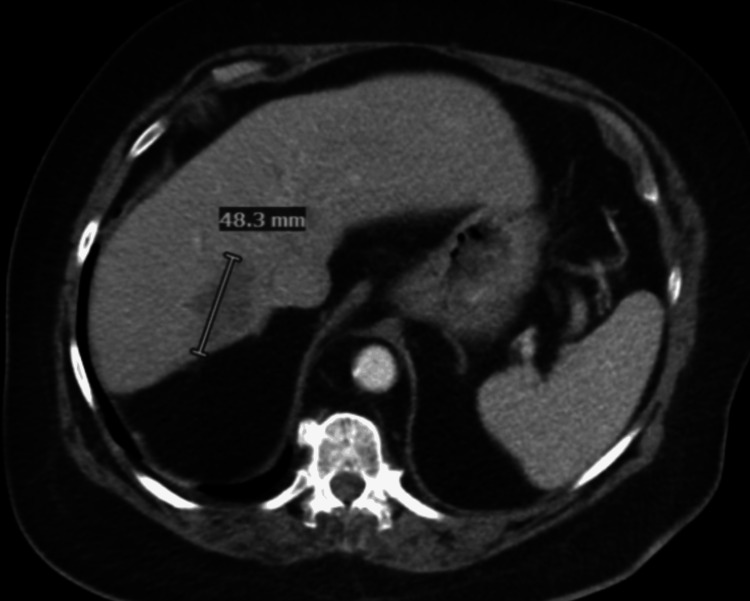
Preoperative abdominal pelvis CT scan with IV contrast visualizing a hepatic lesion in Segment 7.
